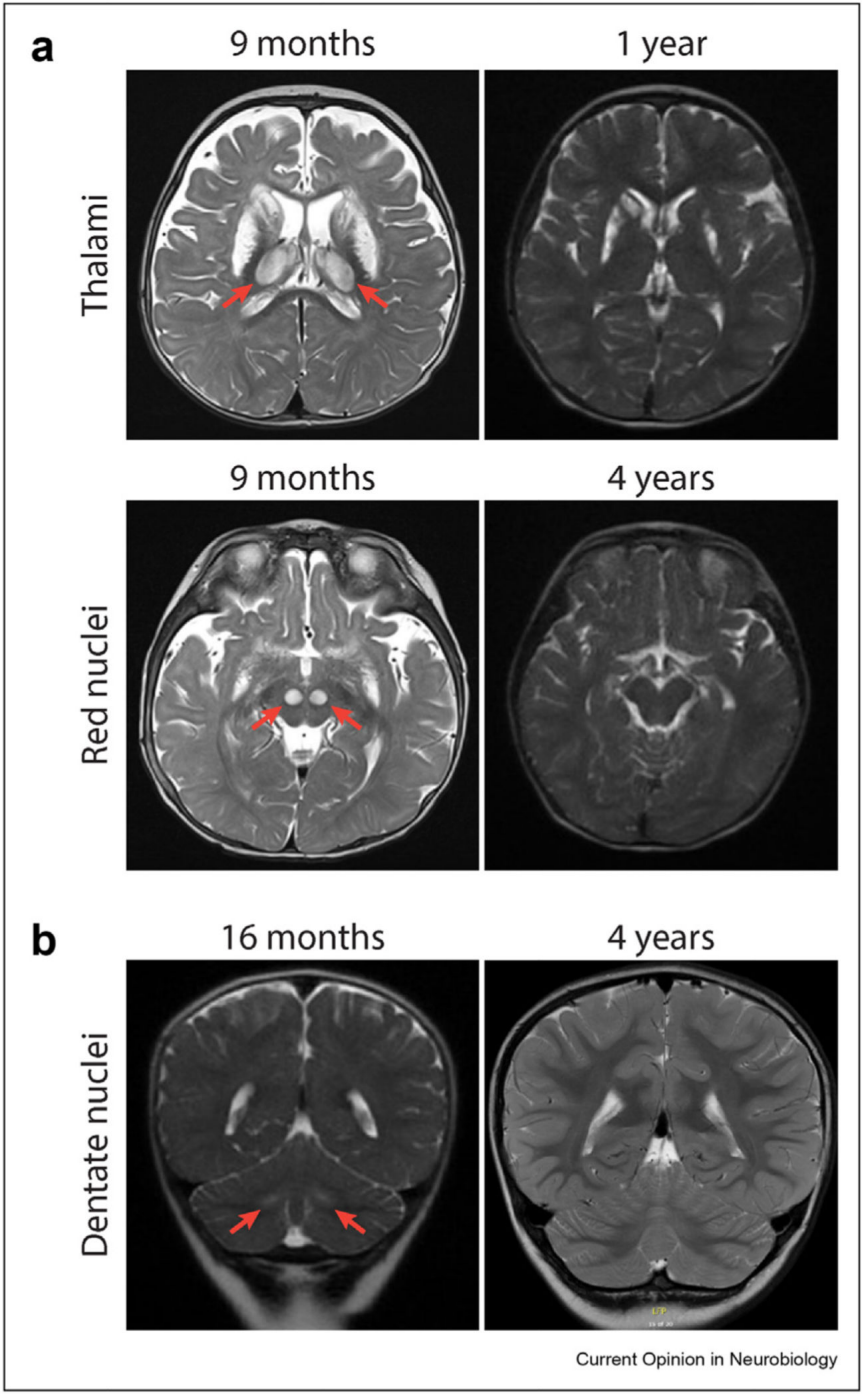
T2-weighted MRI showing resolution of lesions. (a) Patient 1 basal ganglia and thalamic lesions at age 9 months with resolution of thalamic lesions at age 4 years (top) and red nuclei lesions at 9 months with resolution at age 4 years (bottom). (b) Patient 2 demonstrates improvement of dentate nuclei hyperintensities at age 16 months with marked improvement at age 4 years. MRI, magnetic resonance imaging.
